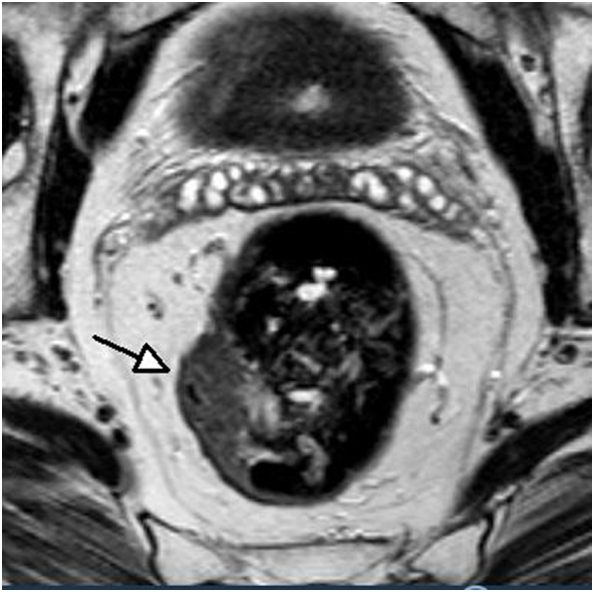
MRI scan at first presentation shows a tumor mass of 2 cm located 11 cm proximal to the anal verge at the right side of the rectum.
